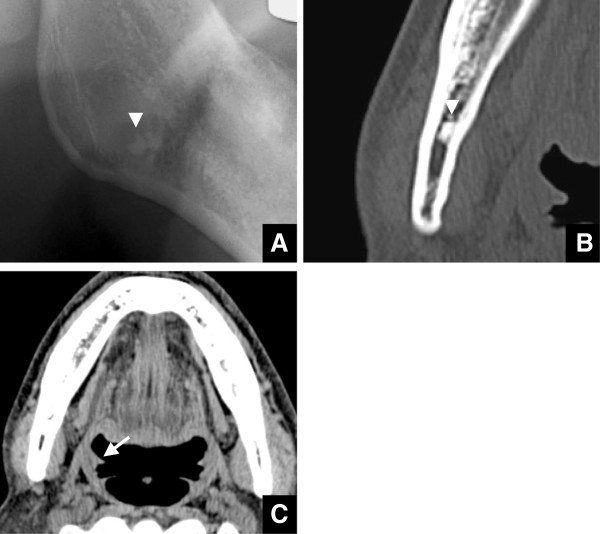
Panoramic radiographs and axial CT images at the palatine tonsillary level of a 78-year-old male. A) A radiopaque nodular mass is shown in the mandibular ramus on panoramic radiographs. B, C) An enostosis in the mandibular ramus (arrowhead), with no tonsilloliths (arrows), is shown as high-density, dot-like structures.
